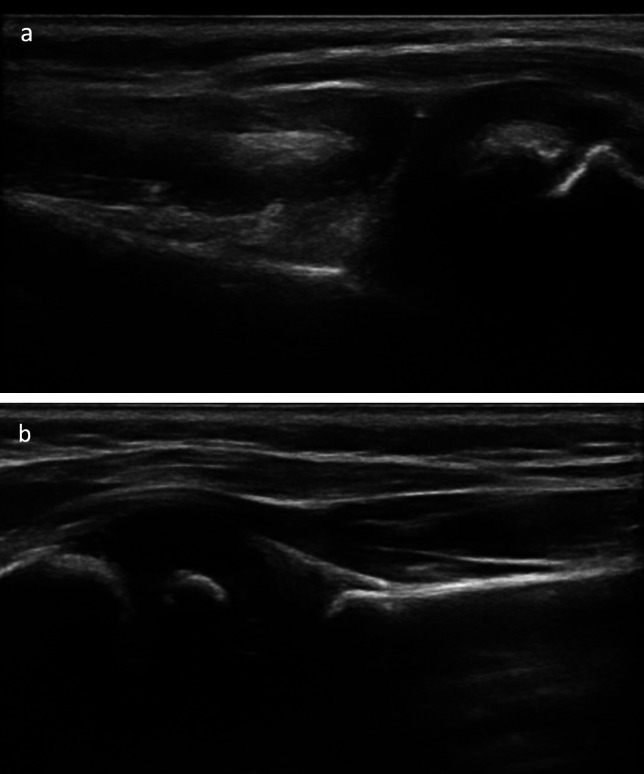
a, b Preoperative ultrasounds of two patients demonstrating dysplastic, ossific nucleus posterior to the scapular line indicative of glenohumeral dysplasia following a brachial plexus birth injury
